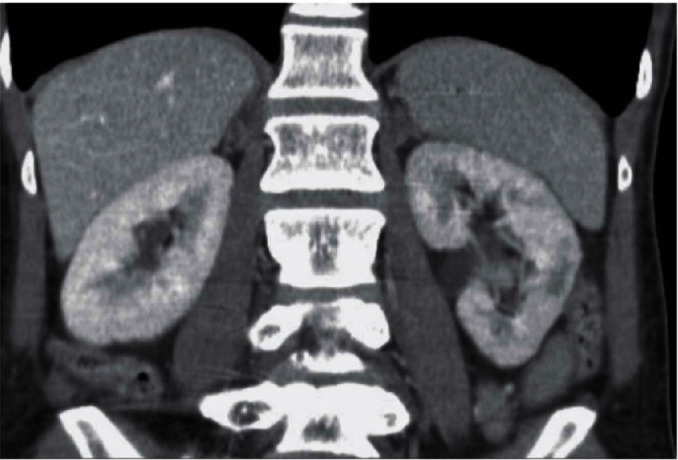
CT angiogram a month later now shows much better renal perfusion with minimal infarcts on the left side, compared to Figure 1.
